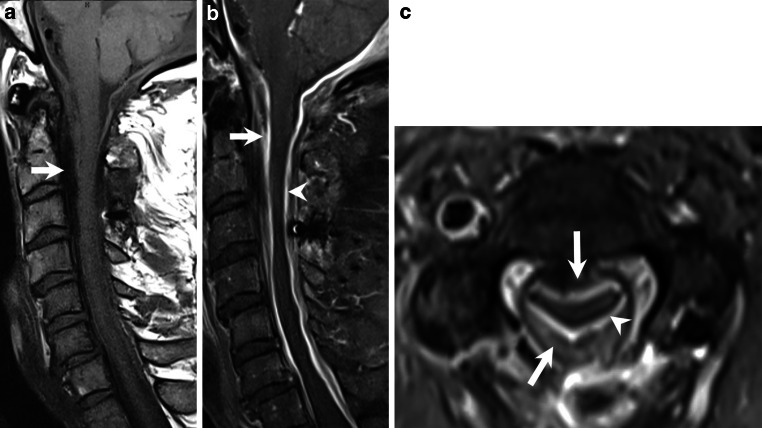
On sagittal native T1-weighted images (a) the space-occupying lesion also appears hypointense (*arrow*). On T1-weighted sagittal (b) and axial (c, level C2/C3) images after administration of gadolinium the meninges display homogeneous enhancement (b, c, *arrowhead*) whereas the space-occupying component shows an irregular enhancement pattern (b, c, *arrow*)
